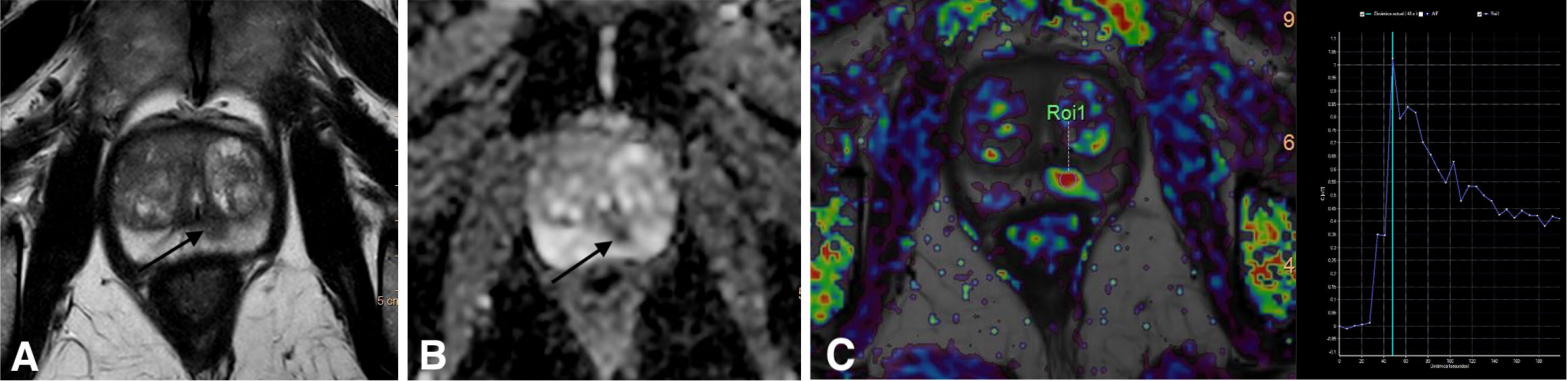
A PI-RADS 4 lesion on the posterior peripheric area of the prostate. (A) Axial T2-weighted sequence: T2 hypointense lesion on the posterior peripheric area of the prostate. (B) Axial diffusion-weighted sequence (ADC maps): focal restricted diffusion lesion. (C) Dynamic contrast-enhanced sequence (functional T1 perfusion maps) with early enhancement and contrast washout of the lesion.
